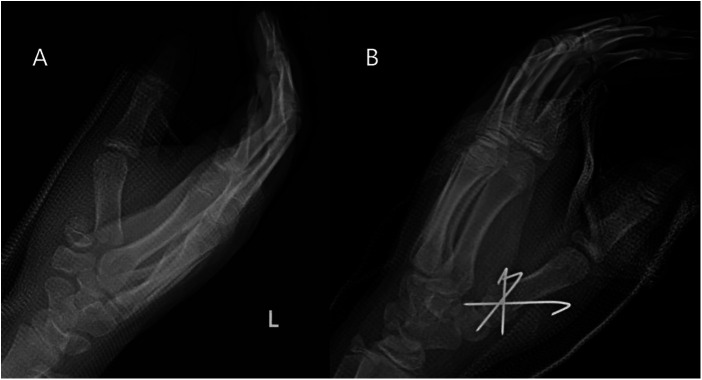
Typical thumb metacarpal base RW-C fracture of 9.3 years old boy. (A) AP x-ray of left thumb preoperative. (B) Result after open reduction with pinning.
